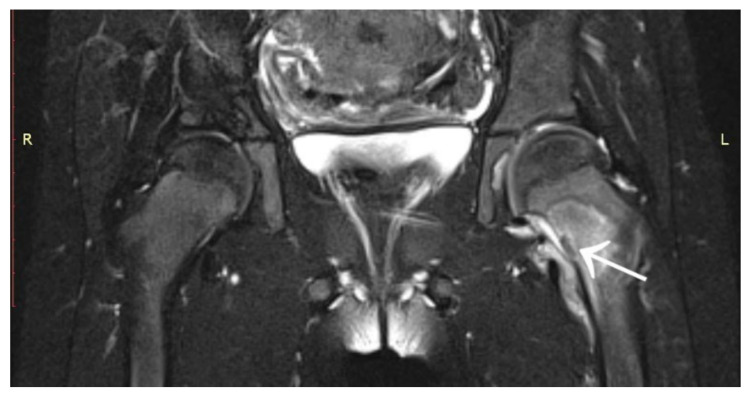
MRI scan showing a focus of OO (arrow) in the left femoral neck.
